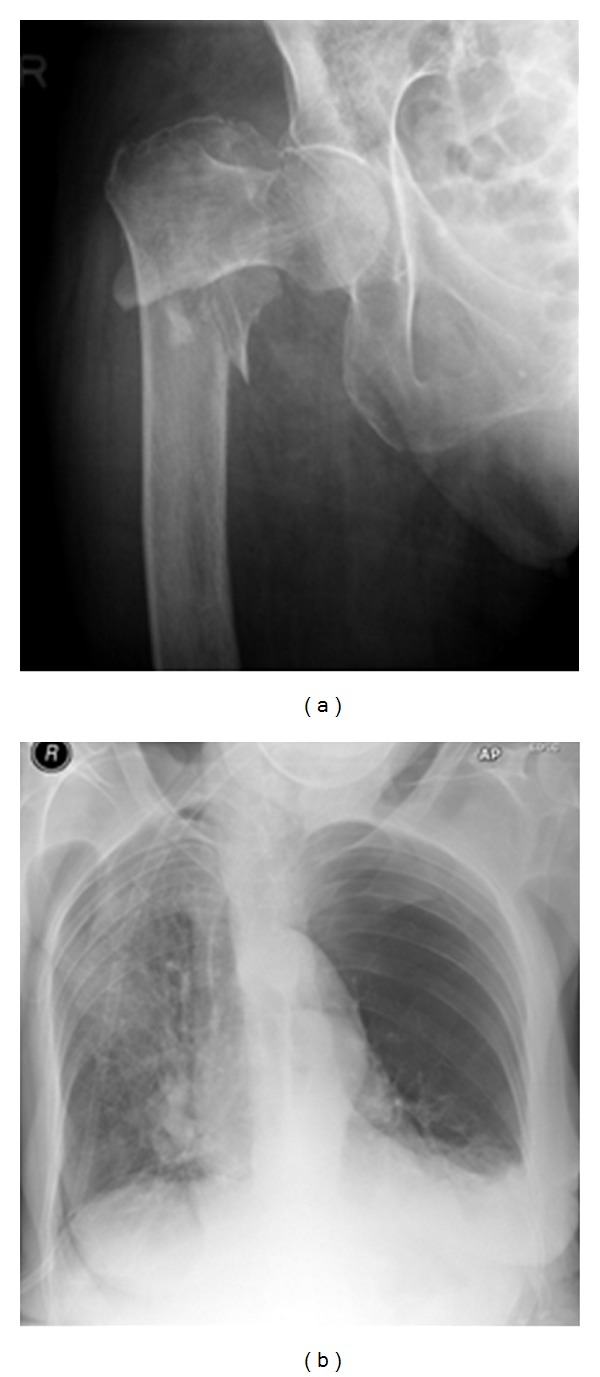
A hip and chest radiograph on an 87-year-old woman following a fall showing a fracture of the neck of the right hip. The CXR was interpreted as showing a left-sided pneumothorax, and thus, a chest drain was put in place (see Figures 25 and 26).
